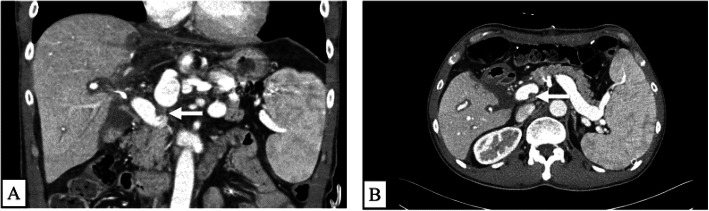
The CT findings of the post-transplant PVT before and the administration of AT III. Post-transplant PVT before the administration of AT III appeared in the left side of the main portal vein at the side of left gastric vein ligation (a and b)
